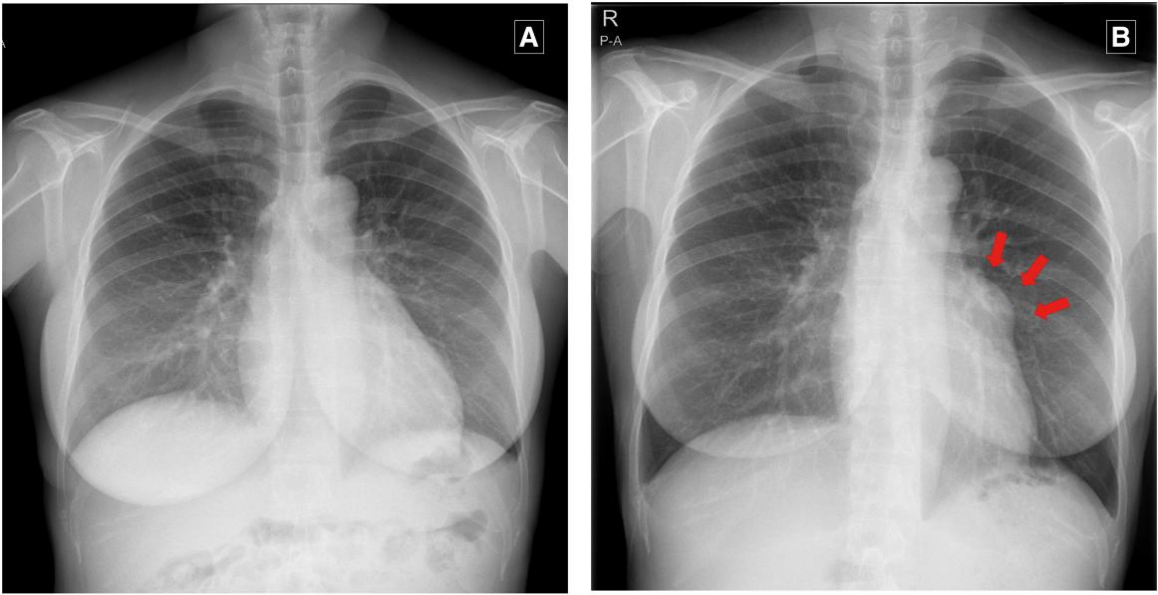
Chest X-ray (*A*) three years previously and (*B*) at presentation. An abnormal silhouette is discernible near the left pulmonary artery (arrows).
